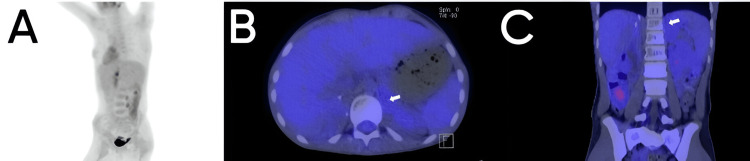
Follow-up with positron emission tomography with fluorodeoxyglucose (PET-FDG) two months after completion of mitotane treatment. Maximum intensity projection (MIP) volumetric images of PET with FDG (right posterior oblique projection) (A); fusion of CT and PET-FDG in the axial plane (B) and coronal plane (C). There is no increase in FDG uptake in the left adrenal gland in the reconstruction in the MIP volumetric images of PET with FDG (A). In the fusion of CT and PET-FDG, it is confirmed that there is no increase in focal FDG uptake (arrow in B and C).
